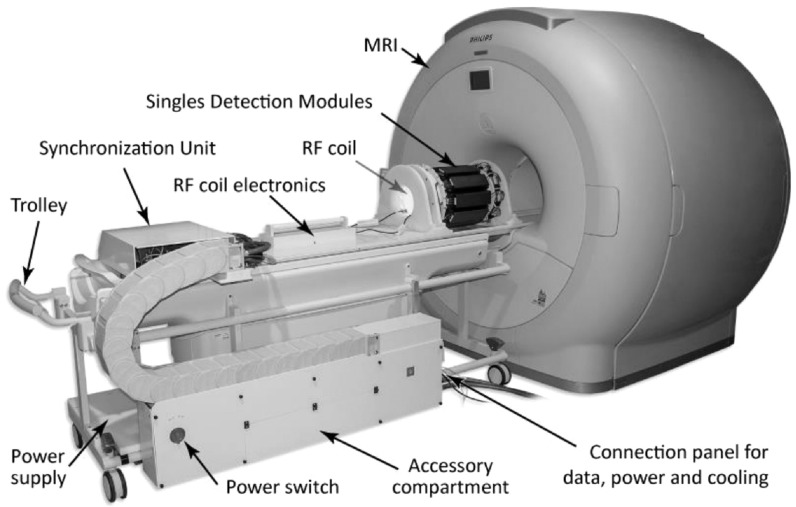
The Hyperion II^D^ PET/MR insert mounted on a patient table in front of a Philips 3-T Achieva MRI system. (Reprinted from (Weissler *et al*
